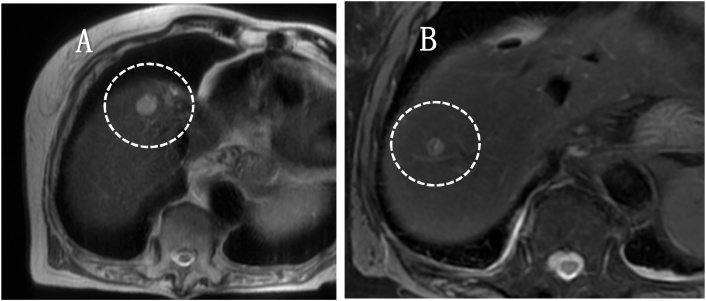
A: The tumour shows a high-signal intensity at segments 3 and 4 on a T2-weighted image in 2017. B: The tumour shows a high signal intensity at segment 8 on a T2-weighted image in 2019.
